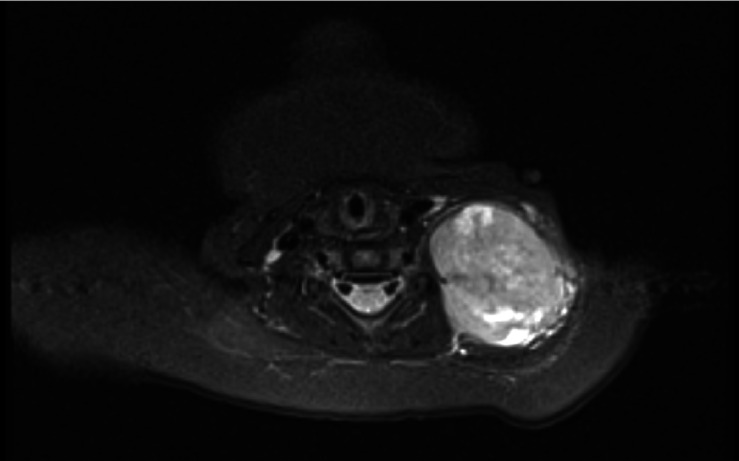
MRI soft tissue neck transverse plane.
